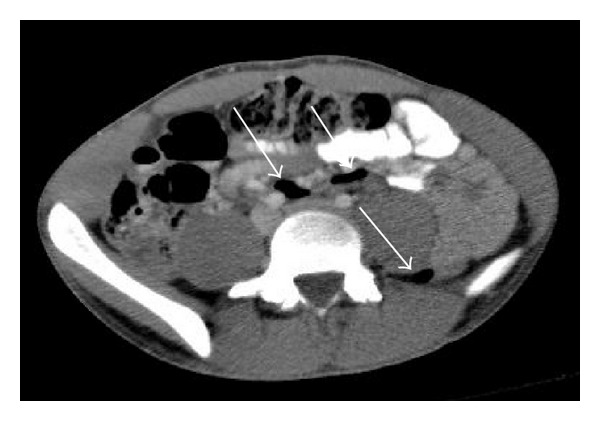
Axial CT demonstrates retroperitoneal air (arrows) anterior to the right and left common iliac arteries, and posterior to the left psoas muscle.
